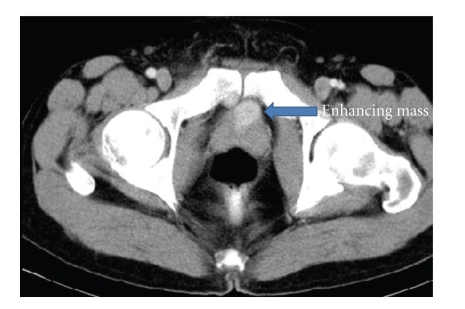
Axial CT scan of the pelvis after IV contrast: oval shape well-defined homogenous small mass at the bladder neck which shows intense enhancement after contrast.
